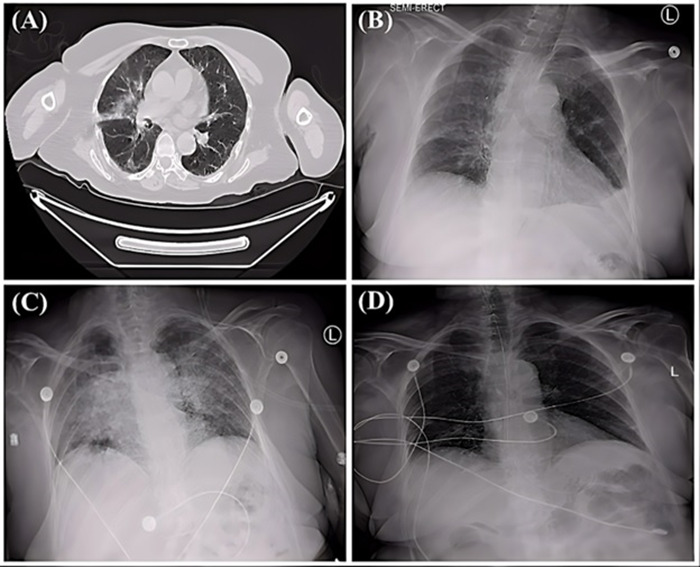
Radiographical findings A. Chest CT revealed bilateral multifocal ground glass opacities notably of the right upper lobe, reactive nodules, and bibasilar reticular densities left more than right. B. Chest X-ray showed infiltration and ground glass opacities. C. Chest X-ray revealed severe bilateral infiltration. D. Chest X-ray showed improvement after bactrim treatment.
